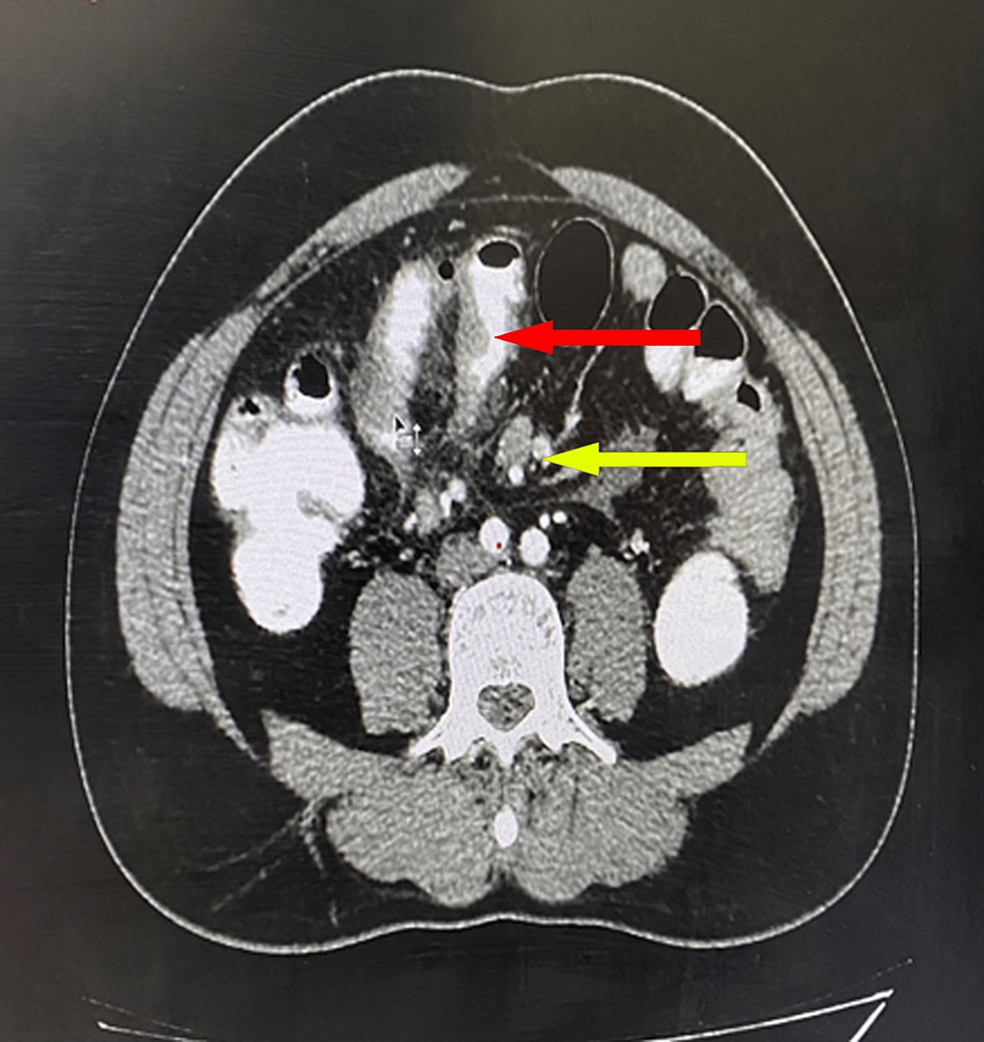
Post-contrast phase axial CT showing long segment edematous thickening of the mid ileal loop with surrounding fat stranding (shown in red arrow), multiple enlarged mesenteric lymph nodes (shown by the yellow arrow), and fluid and adjacent extra luminal air
